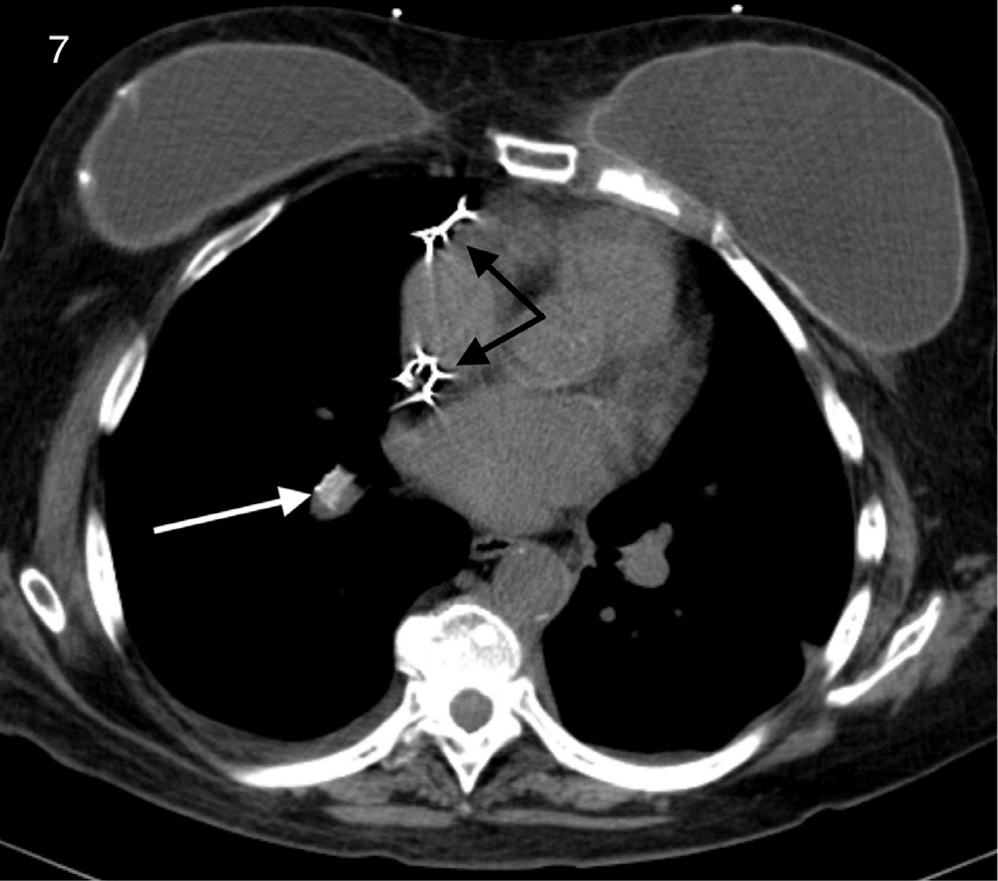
Axial view CT demonstrating the second migrated stent in the right interlobar pulmonary artery (white arrow). Black arrows indicate pacemaker leads placed for complete heart block.
